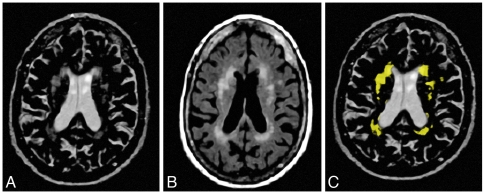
The segmentation process using SNIPER (Software for Neuro-Image Processing in Experimental Research). T2-weighted image (a) and FLAIR image (b). Result of automated segmentation process based on both sequences (c).
